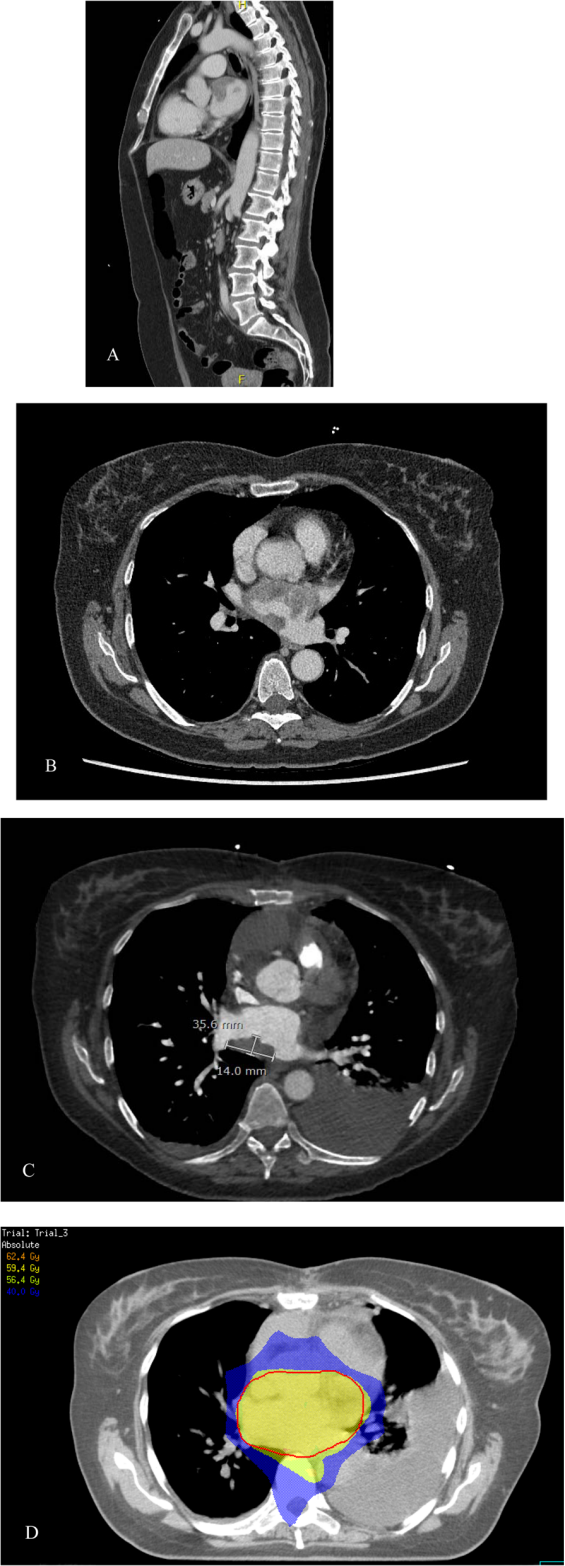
a. CT shows a mass located at the ventral and cranial side of the left atrium expanding into the dorsal site, in sagittal view. b. Transversal view. c. CT made 3 weeks after surgery shows remaining tumor. d. Radiotheraphy planning in transversal view. The red colored line reperesents the planning traget volume
